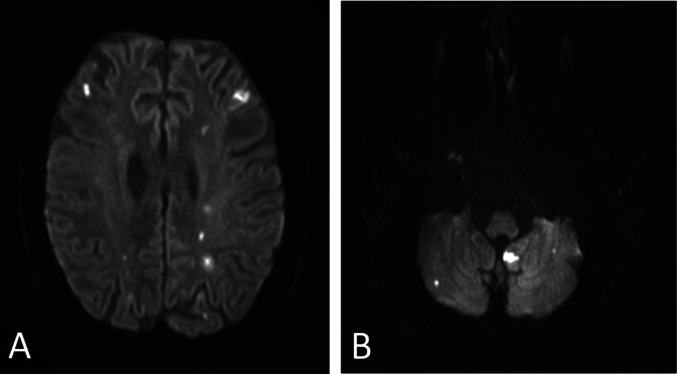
Three-territory sign on cMRI. Acute ischemic stroke lesions on DWI sequence of a patient with infective endocarditis with acute ischemic lesions in the territory of the left and right carotid artery (A) and the vertebrobasilar territory (B).
